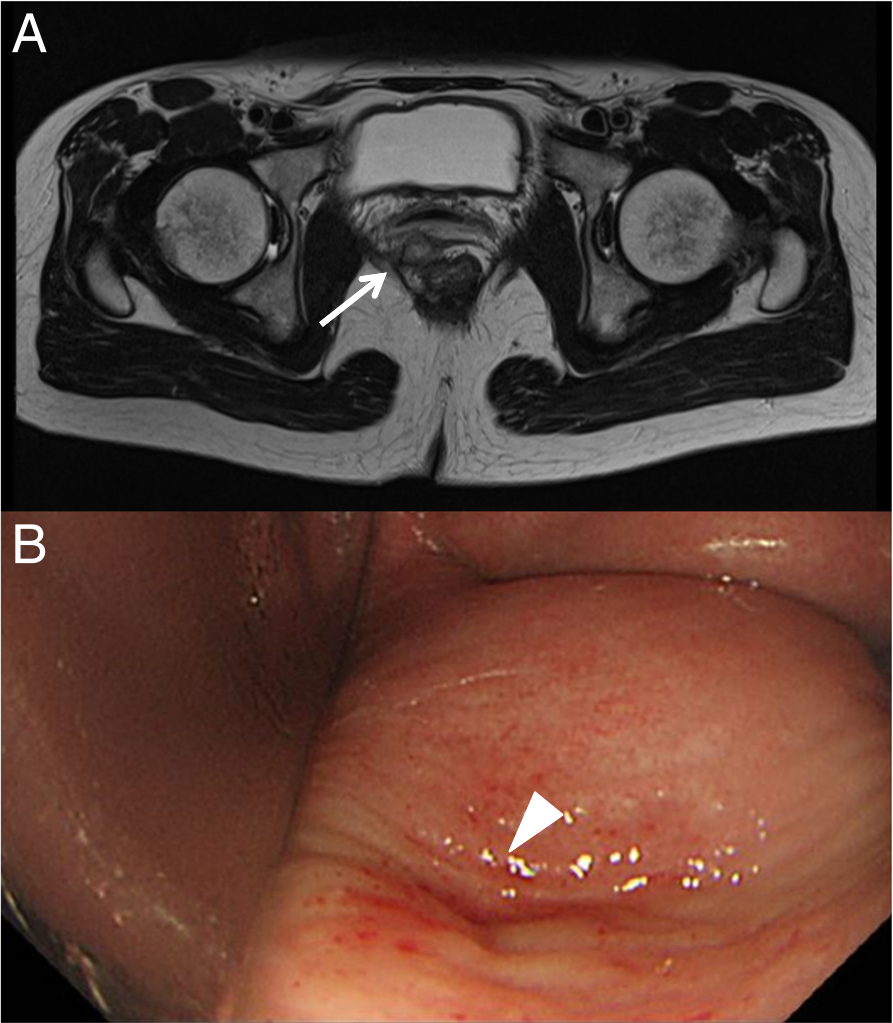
a Case 2. MRI revealed that an anastomotic-vaginal fistula existed at the right anterior side of the coloanal anastomosis (*arrow*). b Case 2. Colposcopy revealed a pin-hole fistula on the posterior wall of the vagina (*arrow head*)
